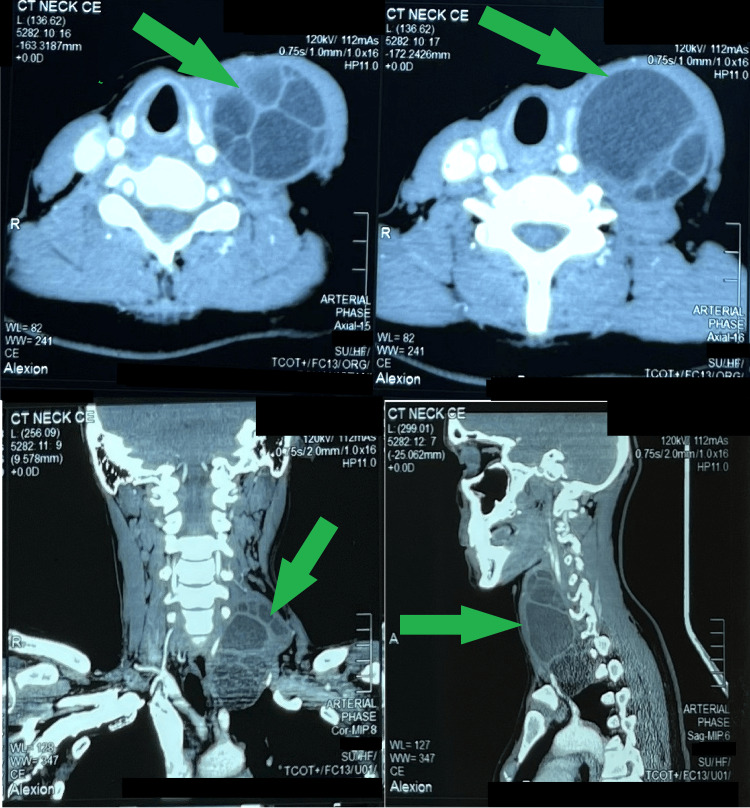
Contrast-enhanced CT images of the neck showing a well-defined multiloculated cystic lesion in the left cervical region with internal septations and daughter cysts (arrows), extending laterally and into the superior mediastinum
